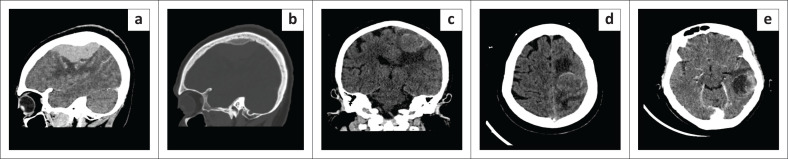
Neoplastic pathology as a cause of adult-onset seizures. (a and b) Meningioma in a 64-year-old female with worsening of left arm weakness and new-onset seizures. Sagittal contrast enhanced CT (CECT). (brain and bone window): Large right frontal avidly enhancing extra-axial mass with associated hyperostosis of the adjacent skull inner table as well as mass effect and oedema of the adjacent parenchyma. (c and d) Left frontal lobe mass in a 59-year-old female who presented with new-onset seizures. Axial and coronal non-contrast CT (NCCT): Large left frontal lobe solid round intra-axial heterogeneously hyperdense mass with surrounding vasogenic oedema and mass effect on the frontal horn of the left lateral ventricle. A differential diagnosis of metastases or lymphoma was given. e: Metastasis from endometroid adenocarcinoma of the endometrium. 69-year-old female with endometroid adenocarcinoma of the endometrium who presented with first onset seizures. Axial CECT: Large ring-enhancing solid-cystic intra-axial mass in the left temporal lobe corticomedullary junction with surrounding oedema.
